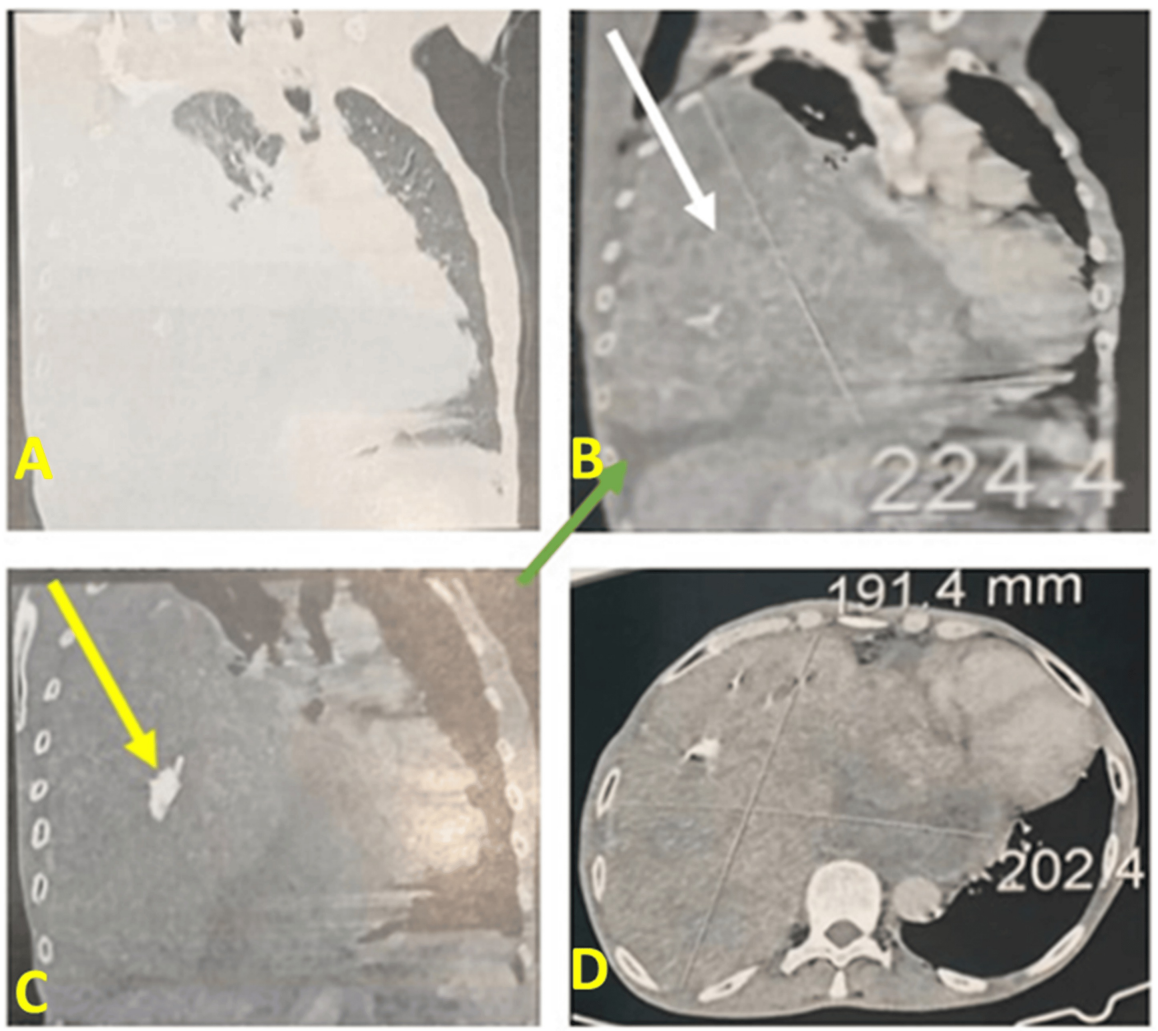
Thoracic computed tomography. (A-D) A large mass (white arrow) in the right posterior inferior mediastinum was measured at 20.2 x 19.1 x 22.4 cm. The mass was heterogeneous in nature, with calcifications (yellow arrow), pleural effusion (green arrow), and mediastinal shift.
